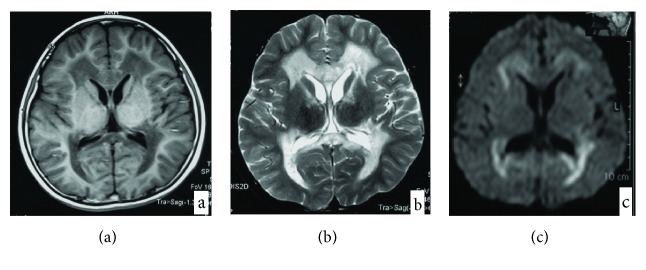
Magnetic resonance imaging (MRI) shows symmetrical deep lesions located in periventricular white matter, which was low signal in T1WI (a), high signal in T2WI (b), and high signal in DWI (c).
